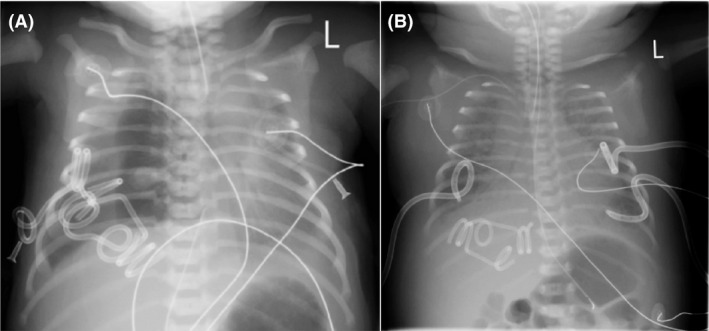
Chest radiograph at birth (A) and prior to pleurodesis (B).
